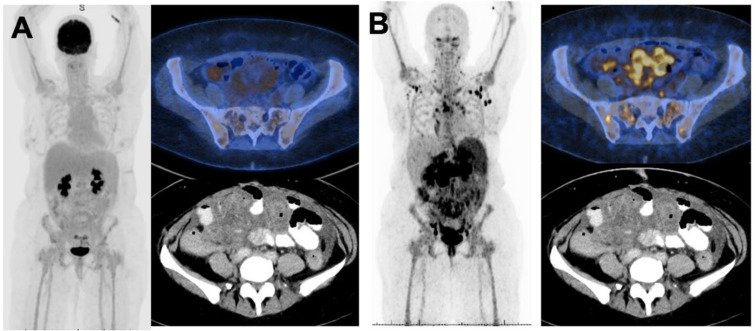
MIP and axial slices from for [^18^F]FDG PET-CT (A) compared to 1 h [^64^Cu]Cu-SAR-BBN (B) in Patient 5 shows bony, nodal and bowel serosal disease was BBN avid but FDG negative.
